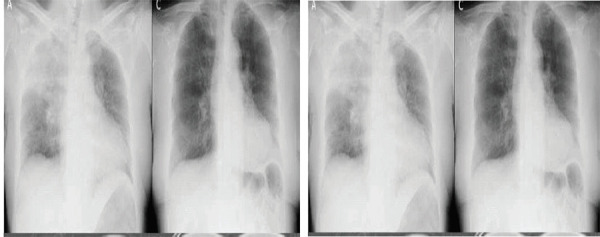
X-ray taken after reintubation and after 4 hours.
